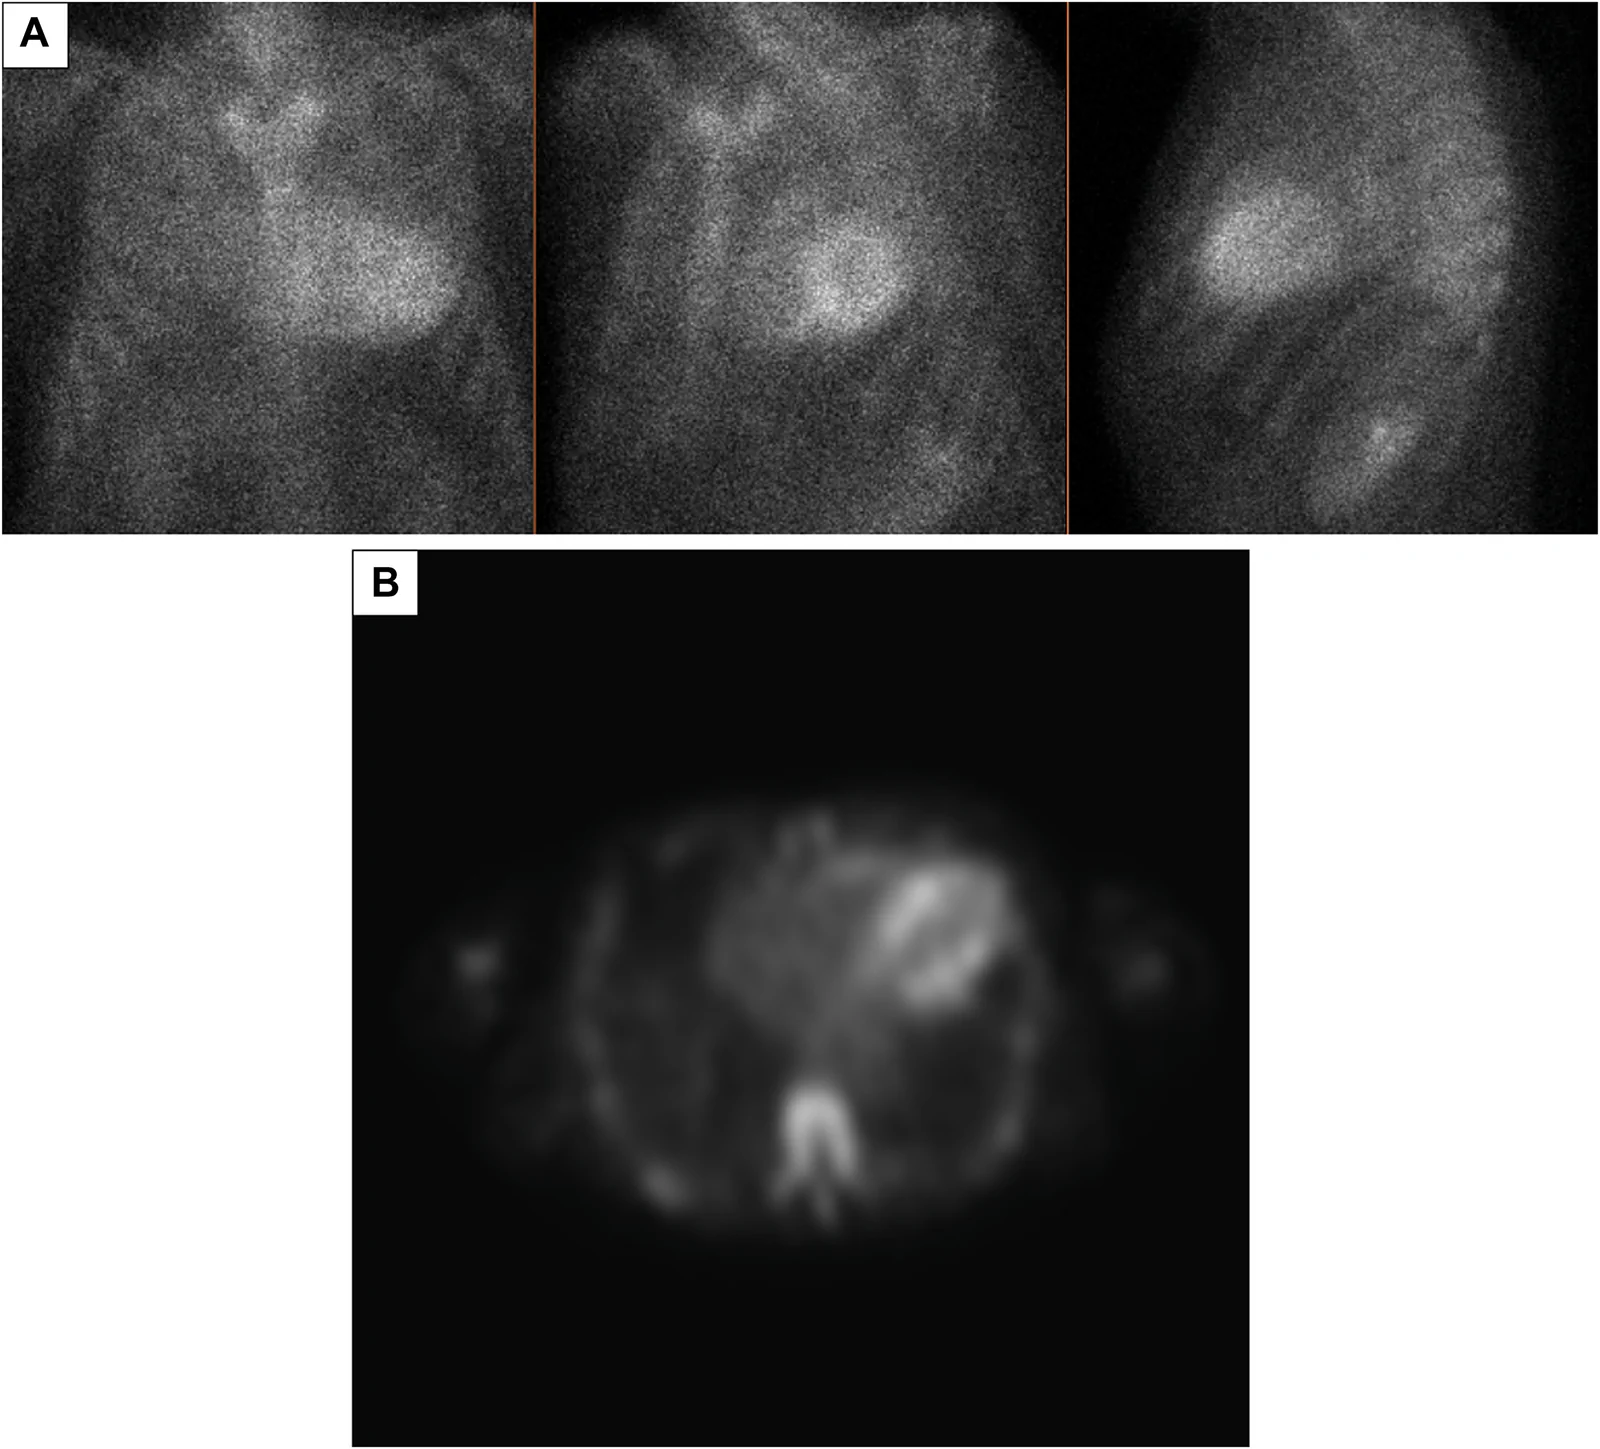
Technetium 99m Pyrophosphate Imaging (A) Diffuse myocardial uptake on anterior, left anterior oblique, and lateral views. (B) Single-photon emission computed tomography image showing diffuse myocardial uptake isolated from blood pool.
